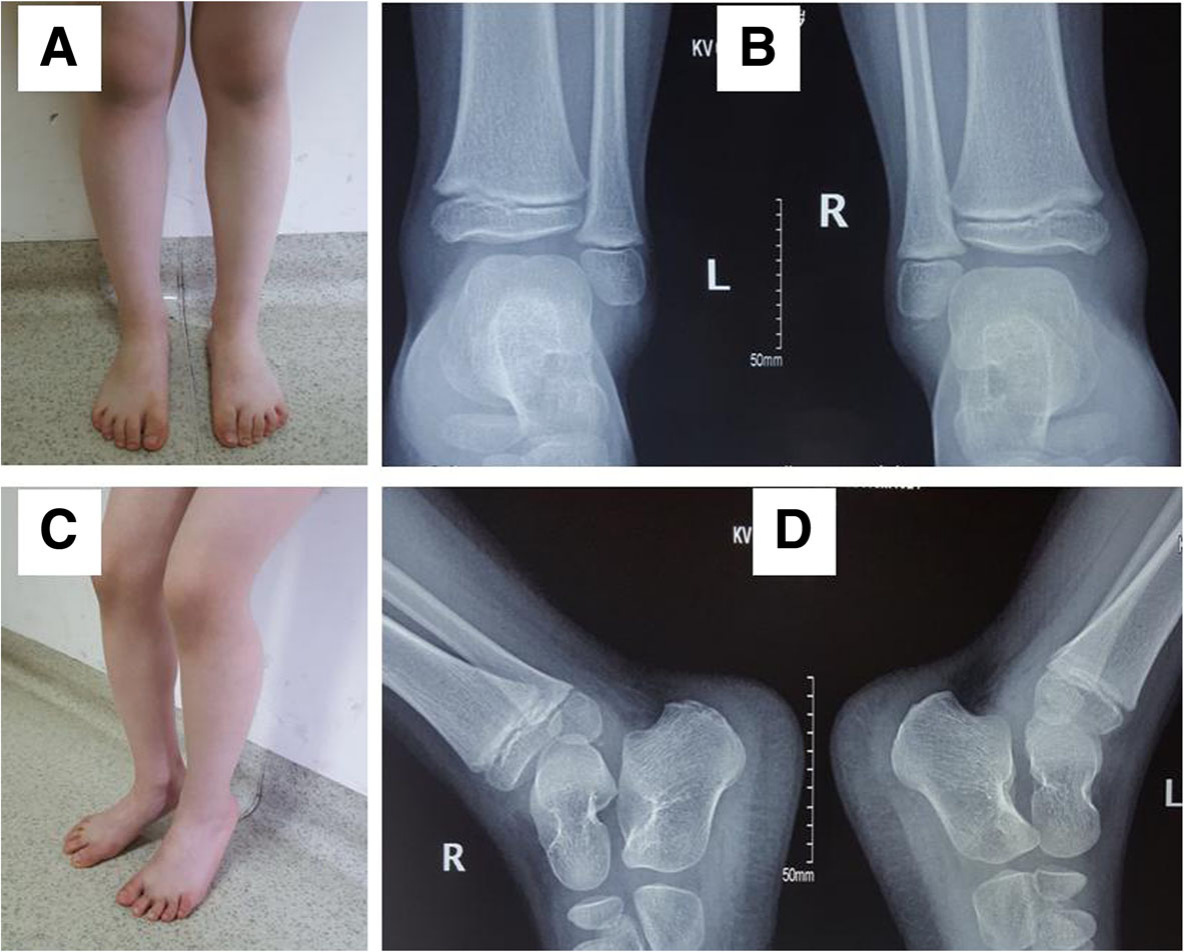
General observation (a, c) and X-rays (b, d) of the patient in Fig. 1 at postoperative 2 years indicating that the fracture had healed and ankle joint function had restored
